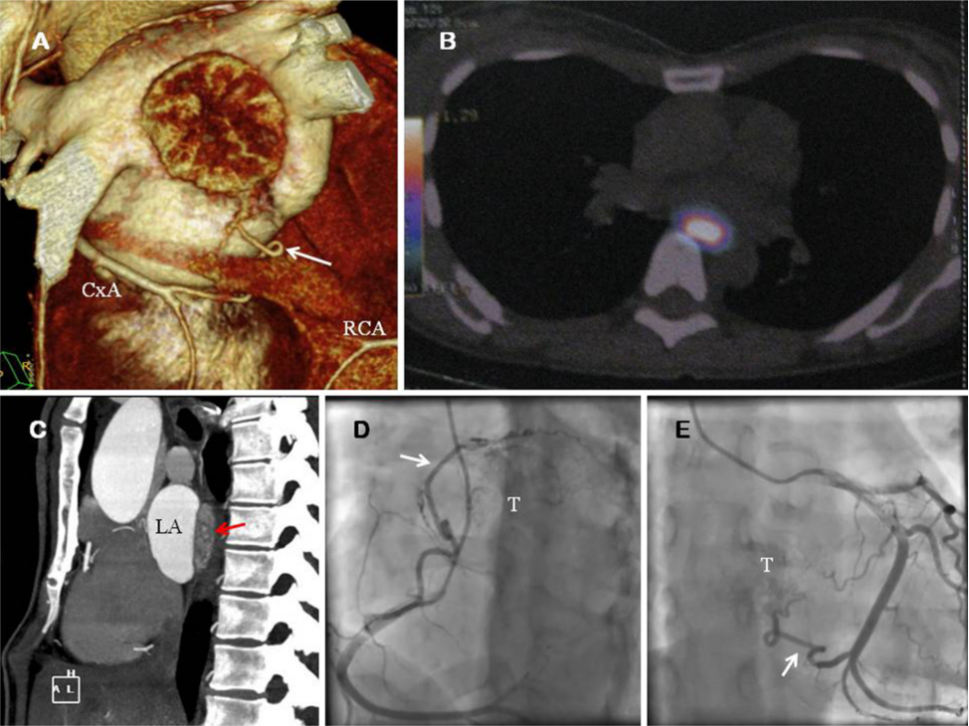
A. Computed tomography imaging reconstruction of the heart. The tumor is showed. The collateral vessel (white arrow) from the circumflex artery (CxA) is observed. B. 18-F DOPA PET showed a 16 × 25 × 36 mm extracardiac mass protruding within the LA. Presence of metabolic activity was detected. C. Computed tomography (axial view) showed the presence of the large mass (red arrow). D. Coronary angiography. The coronary blood supply of the superior aspect of the paraganglioma (T) emerged from a large collateral vessel (white arrow) from the right coronary artery (RCA). E. Coronary angiography. Proximal and distal collaterals from CxA were the feeding vessels (white arrow) of the inferior aspect of the tumor (T).
